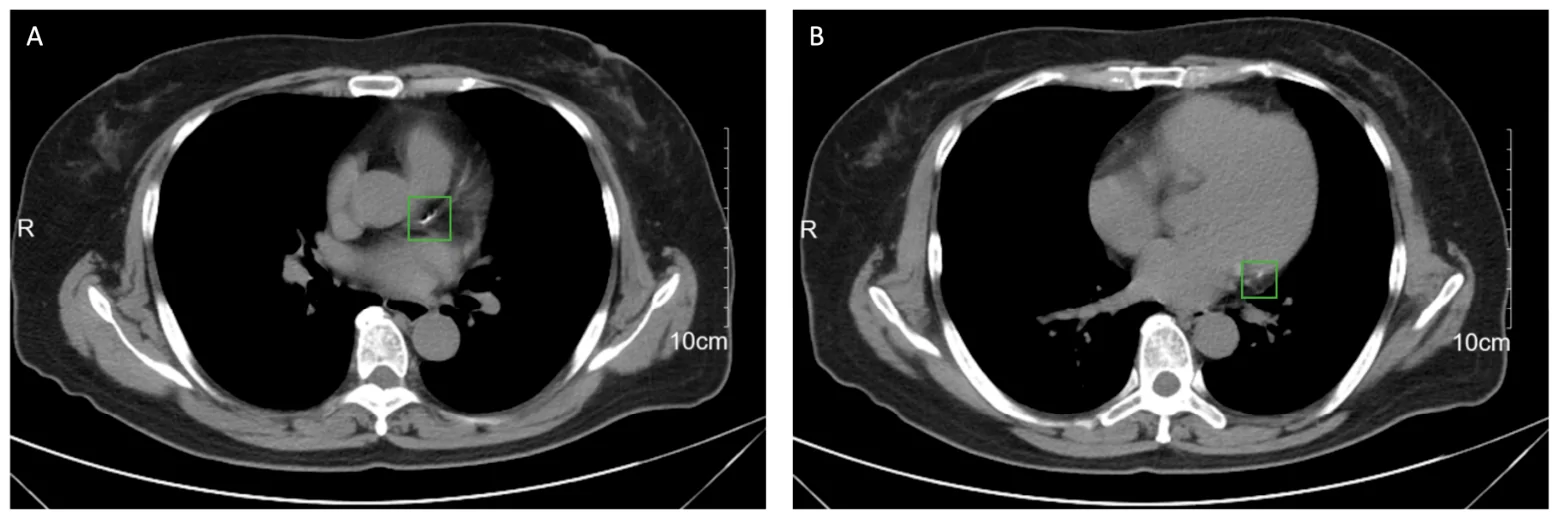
Preoperative chest-computed tomography. (A) Calcification in the left anterior descending artery (green box). (B) Calcification in the left circumflex artery (green box).
